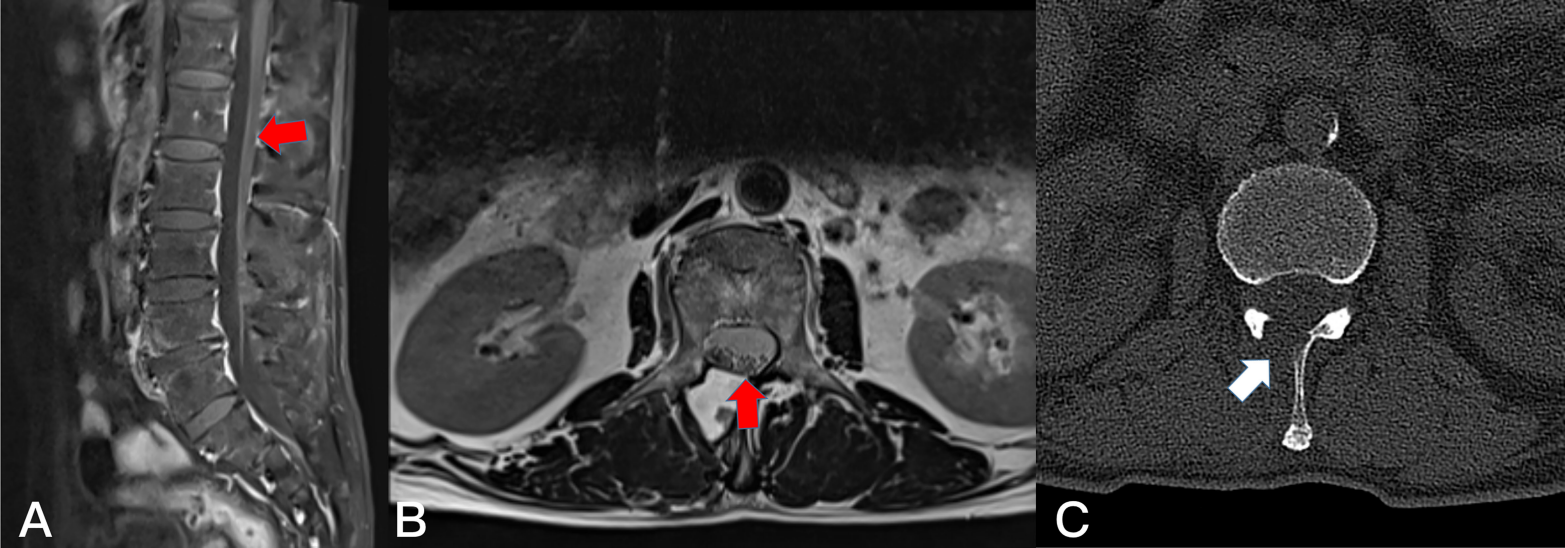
Postoperative T1-weighted enhanced MRI on sagittal (A) planes and T2-weighted MRI on axial (B). Resection of the subdudral tumor could not be identified after 1 months (red arrows). The axial plane (C) of CT scan showed the bone window of L1 lamina (white arrows).
